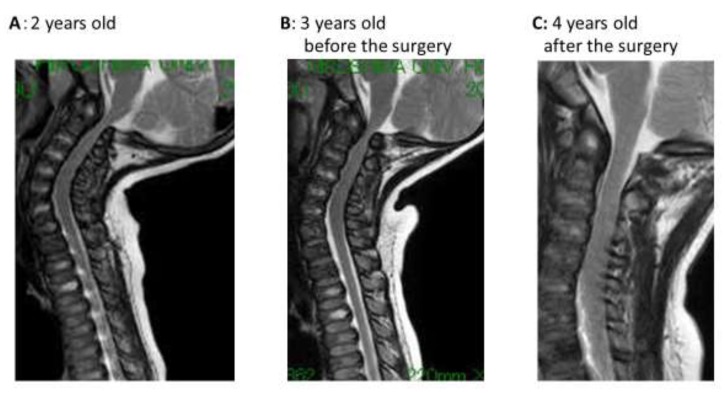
The changes in MRI findings. (A) The spinal cord compression at C1 level was already present at the first examination. (B) The lesion was slowly progressive after the ERT started, (C) which was relieved by resecting the posterior arch of the atlas vertebra.
